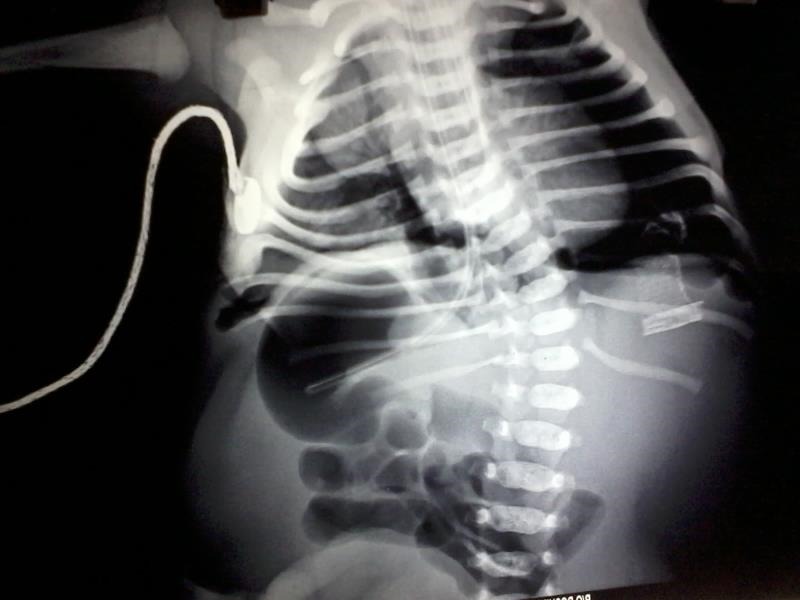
Figure 2: X-ray showing left pneumothorax, right lung hypoplasia, liver on left, stomach bubble on right, floating ribs and scoliosis.
